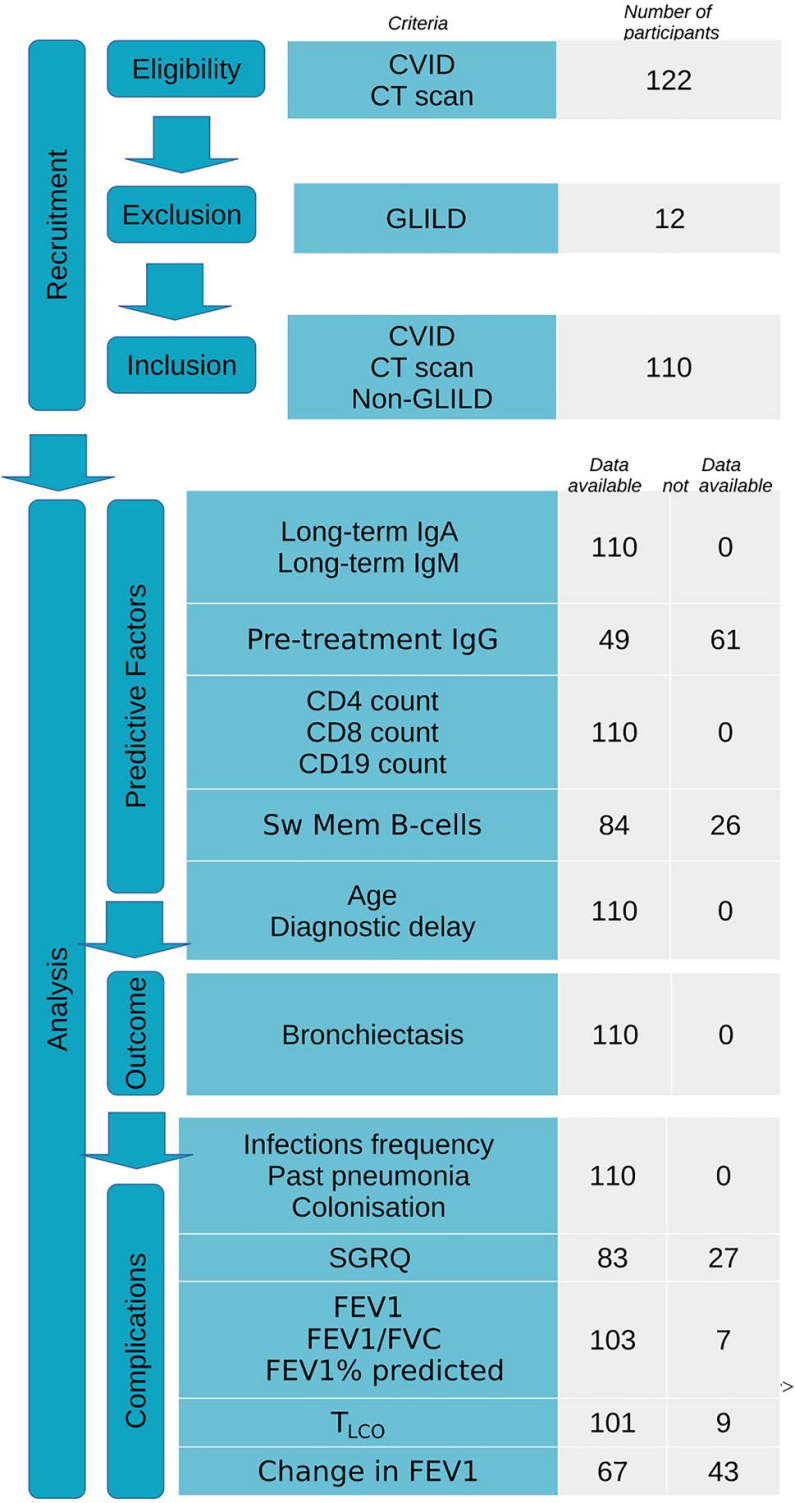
Participants and variable chart. 122 patients with CVID and a CT scan were eligible for our study. We excluded 12 patients with GLILD. Immunological parameters, age and diagnostic delay were independent variables to establish predictors for bronchiectasis. CT scans were assessed for the presence of bronchiectasis (dependent variable). Infection history, quality of life, and lung function were used to compare complications in patients with and without bronchiectasis
